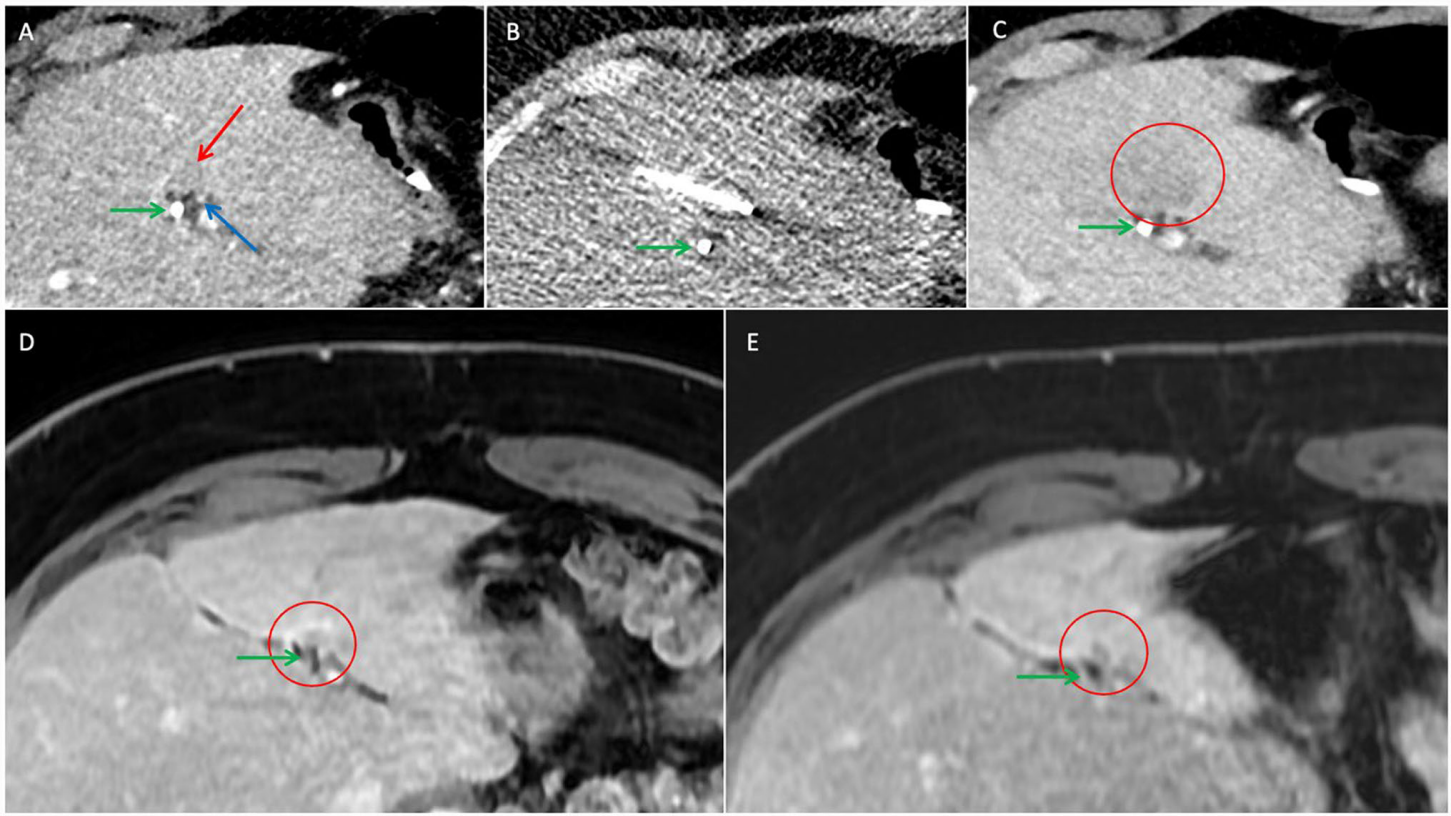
CT-guided cryoablation of patient 3 with a NET metastasis near the left hepatic duct and follow-up (2 and 11 months) CTs. A: Pre-ablation planning CT shows a 9-mm hypodense NET next to the left hepatic duct with a minimum distance of 3 mm. B: Position of the cryoprobe just before ablation. C: Post-ablation imaging shows complete necrosis of the HCC with inflammatory rim enhancement. D, E: The ablation zone continuously is shrinking with reduced inflammatory rim enhancement at the 2- and 11-month follow-up, indicating no residual or recurrent tumor. *red arrow: tumor; *red circle: post-ablation necrosis; green arrow: bile duct; blue arrow: hepatic artery. *NET: Neuroendocrine tumor
